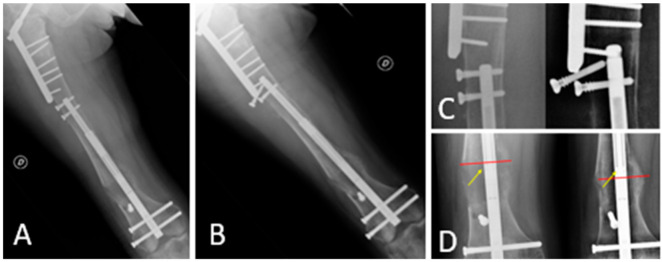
(A): Follow-up X-ray conducted 2.5-month postoperatively; (B): follow-up X-ray conducted 3-month postoperatively showing loosening of the proximal anchoring screw; (C): the upward migration of the nail, complete loss of the proximal screw, and partial loss of the other proximal screw, with the cortical bone that eroded; (D): the decreased height of the bone regeneration (Indicated by the red line), which is below the magnetic component (Indicated by the yellow arrow).
